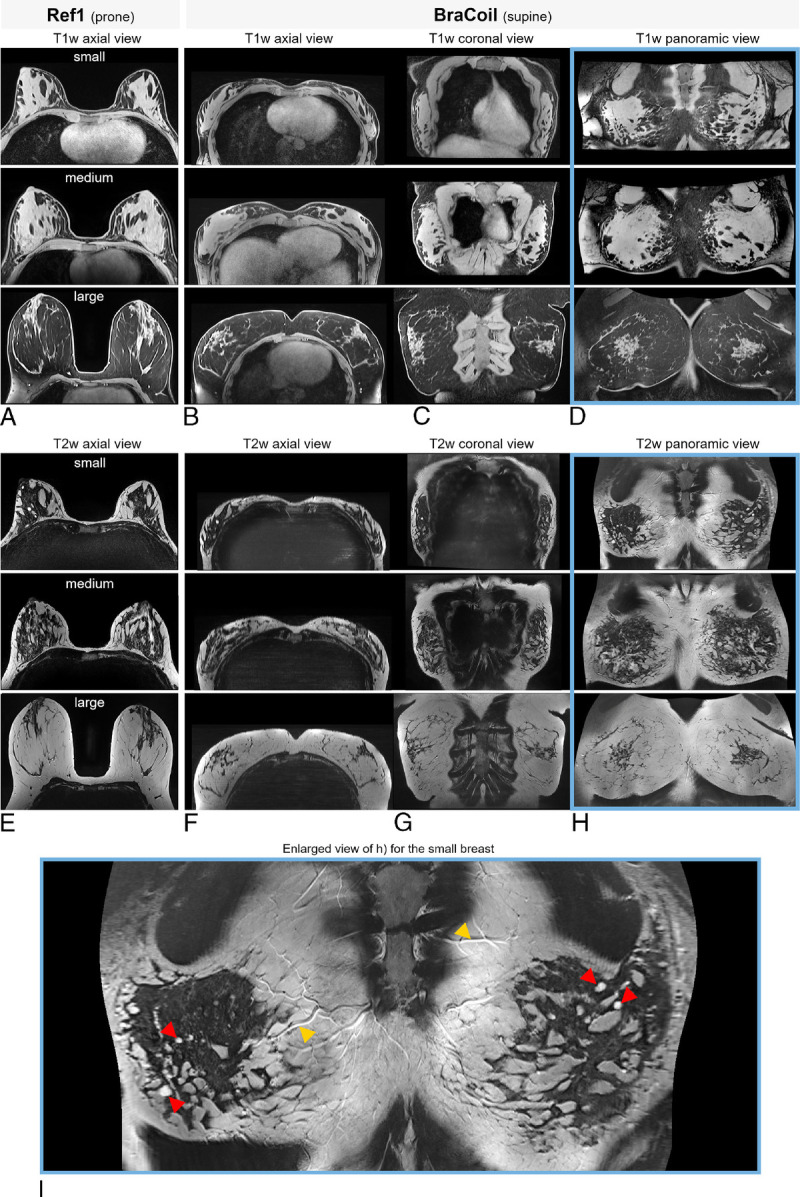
T1- and T2-weighted images from healthy volunteers. The potential of panoramic breast MRI is demonstrated on healthy volunteers. Excellent image quality can be observed throughout the data, and the intuitive nature of the panoramic view can be appreciated. T1-weighted (A–D) and T2-weighted (E–I) images are shown for subjects with small (657 mL), medium-sized (1338 mL), and large (3020 mL) breasts. Panoramic breast images are highlighted by blue borders. The left column (A, E) shows data acquired with Ref1 “16-channel Sentinelle Breast Coil” in the prone position. The other columns (B–D, F–I) show supine BraCoil data. For comparison to the reference images, in the second column (B, F), the axial view of the supine MRI data is shown. Column 3 displays the same data in a coronal view (C, G). In column 4, the proposed panoramic view of supine breast MRI data is presented. Panel I shows an enlarged version of H for the small breast to demonstrate the image quality. Red arrows, benign uncomplicated cysts; yellow arrows, vessels.
